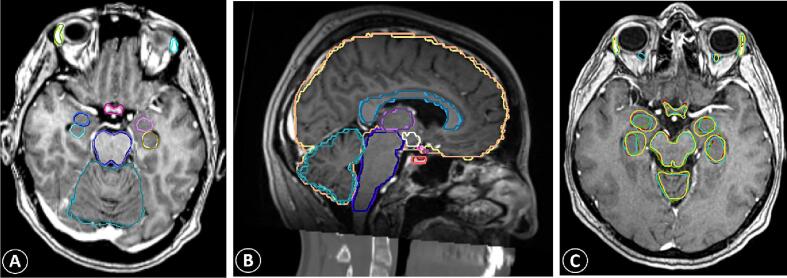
A, B- Example case with reference structures in darker and AI-generated structures in lighter shades. C: Axial MRI showing inter-observer variability between clinicians (yellow and green), reference structures (cyan) and AI contours (red).
